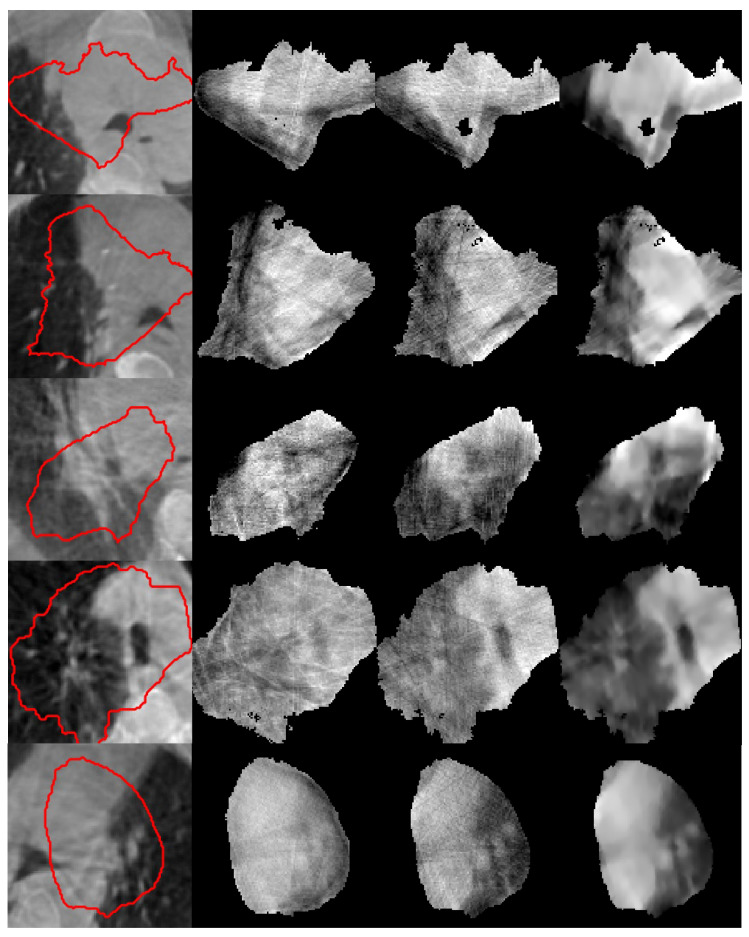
VMAT-CT reconstructions of real-patient cases. (First column) Pretreatment CBCT images overlaid by the prescription isodose lines (red); (second column) VMAT-CT reconstructed with FDK; (third column) VMAT-CT reconstructed with FDK + preprocessing; (fourth column) VMAT-CT reconstructed with iterative + preprocessing.
